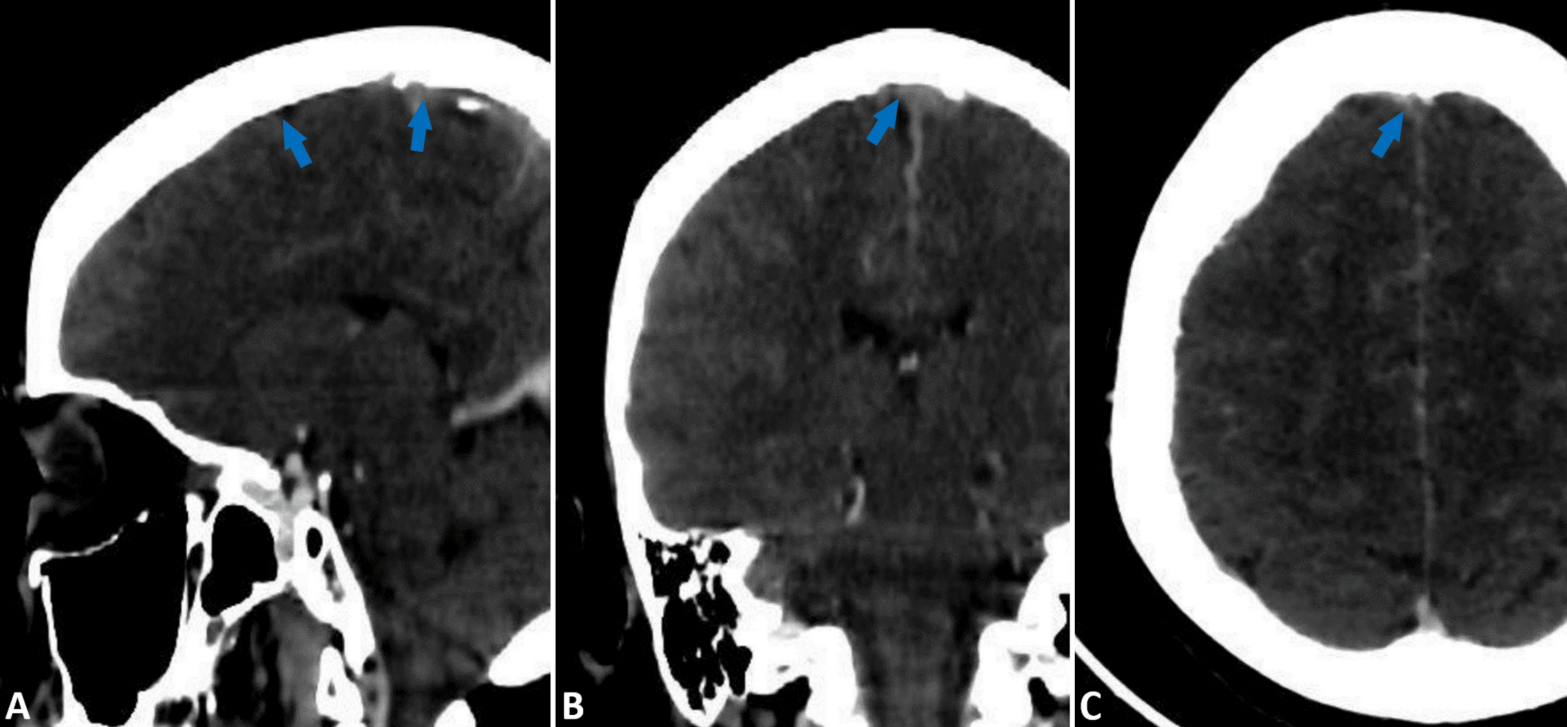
Contrast-enhanced cerebral CT scan in sagittal (a), coronal (b), and axial (c) views demonstrates the absence of opacification in the superior sagittal sinus, indicative of cerebral venous thrombosis (Blue arrows).
